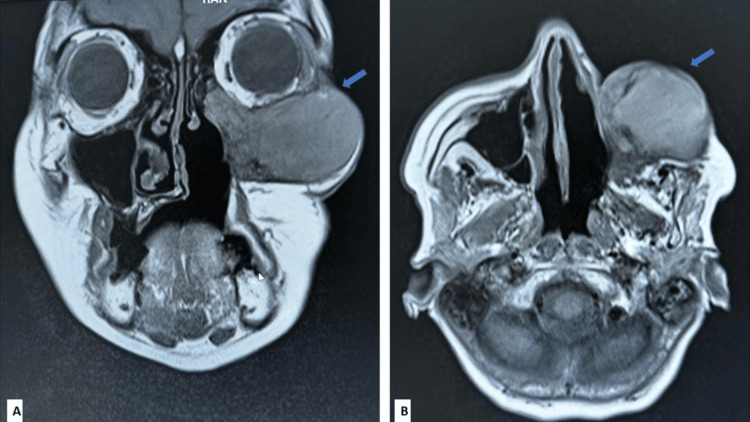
Magnetic resonance imaging (MRI) of the paranasal sinuses and orbit Magnetic resonance imaging (MRI) of the paranasal sinuses and orbit demonstrating a mass-forming lesion. A. Coronal section shows a heterogeneously intense soft-tissue mass occupying the maxillary sinus with extension into the adjacent nasal cavity and infraorbital region, causing facial asymmetry (blue arrow). B. Axial section reveals the lesion abutting the orbital floor with mass effect on the orbit, mimicking a neoplastic process (blue arrow).
